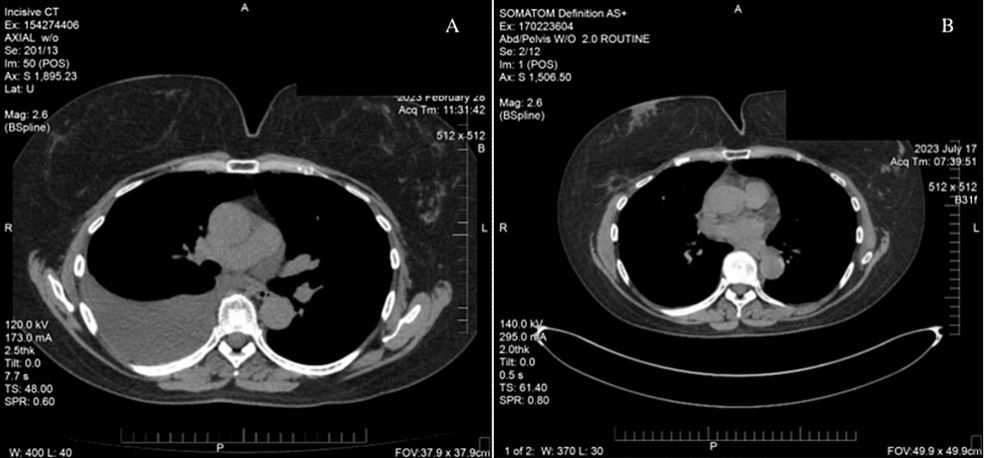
CT scan images showing right-sided pleural effusion resolution (A) At symptomatic presentation on February 28, 2023; (B) showing complete resolution on July 17, 2023.
